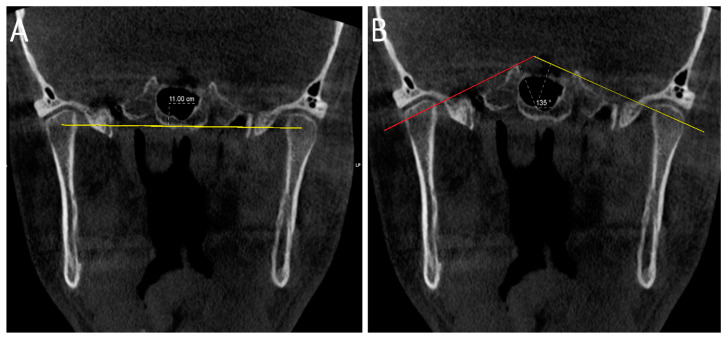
Coronal reformatted CBCT images of a patient showing measurement of the intercondylar angle and distance. In image (A), the coronal intercondylar distance equals the yellow line joining the centre of the right and left condyles, In Image (B), the coronal intercondylar angle is the angle between the lines joining the medial and lateral pole of the right condyle (red line) and the line joining the medial and lateral poles of the left condyle (yellow line).
